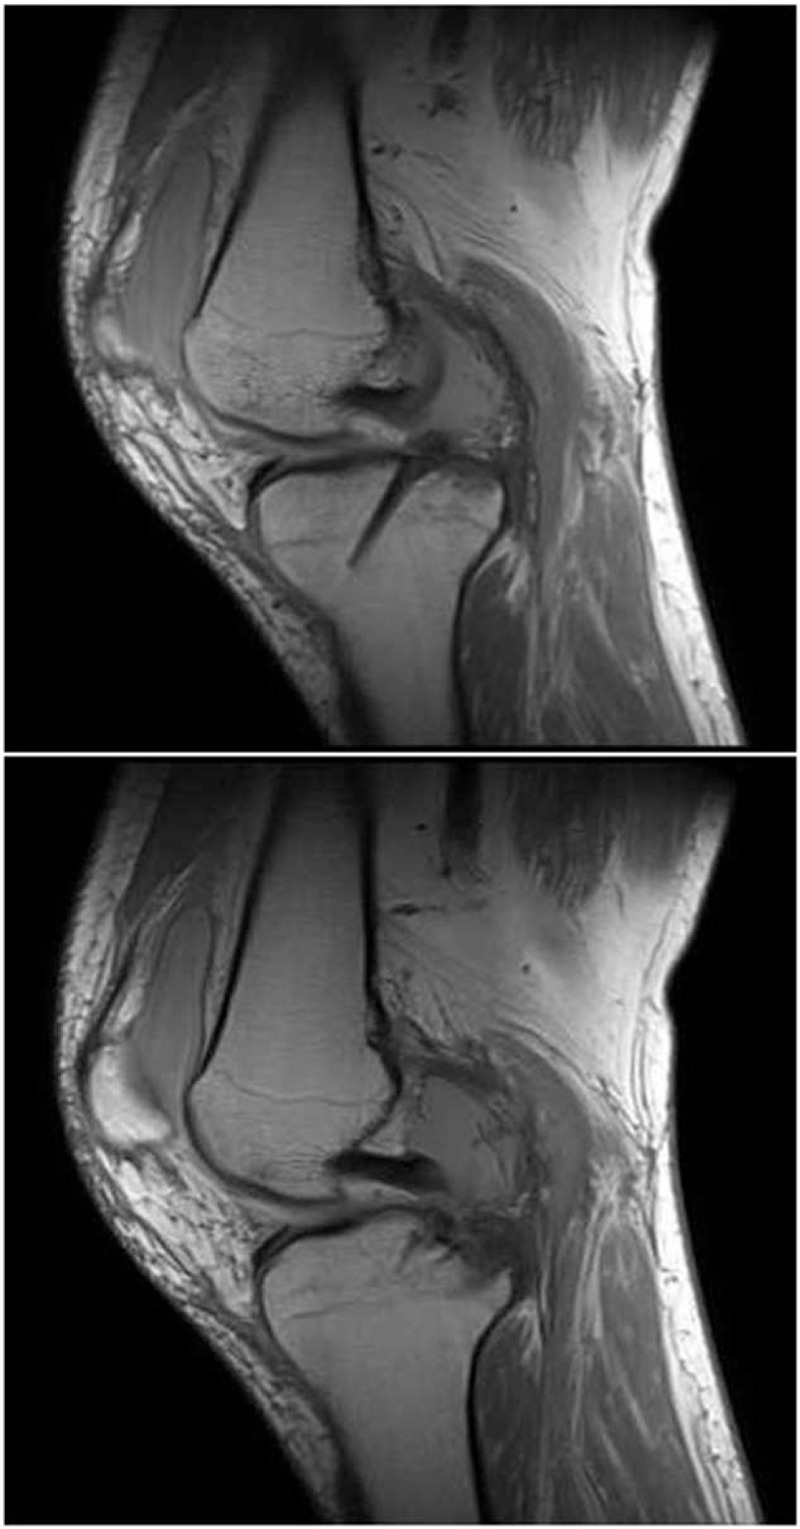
MRI 5 months after the initial operation revealing that the position of the absorbable screw was more anterior and medial than normal.
